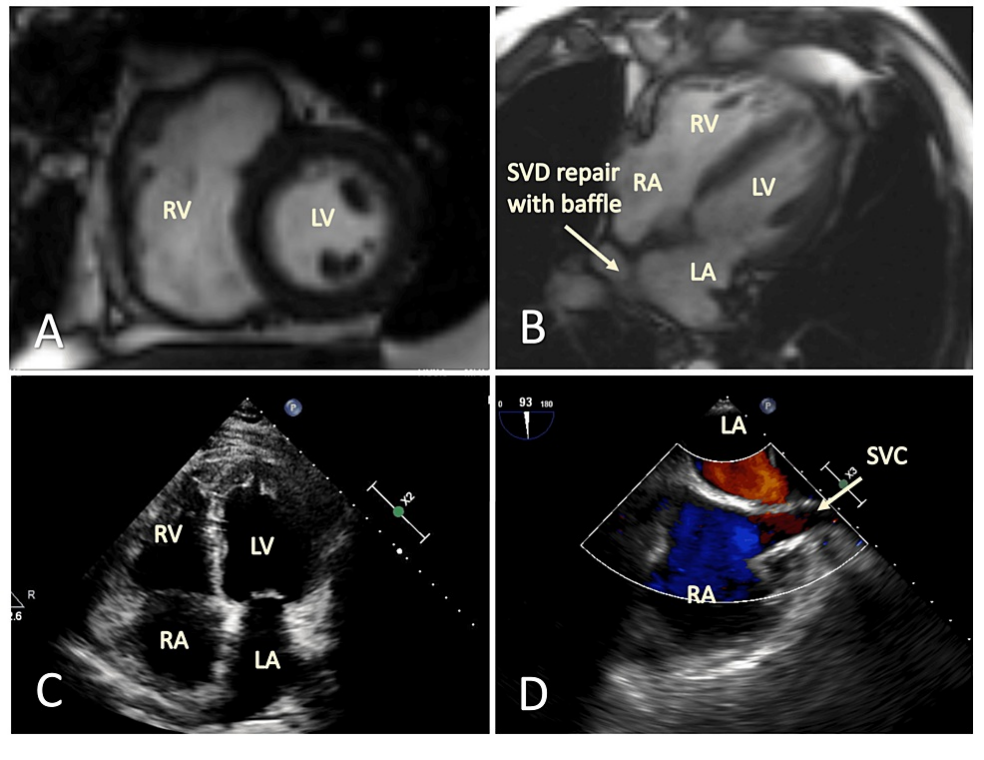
Follow-up images after surgical repair of the RSAPVR and SVD CMR images on follow-up; (A) Demonstrates an improvement in RV dilation. (B) The surgical correction of the SVD and RSAPVR repair with a baffle conduit can be appreciated, restoring the right superior pulmonary vein into the LA. (C) TTE images in the apical 4-chamber view demonstrate improved RV dilation. (D) TEE image of the bicaval mid-esophageal view demonstrates resolution of the inter-atrial shunt. CMR: cardiac magnetic resonance imaging; RV: right ventricle; RA: right atrium; LV: left ventricle; LA: left atrium; SVD: sinus venosus defect; SVC: superior vena cava; RSAPVR: right superior anomalous pulmonary venous return; TTE: transthoracic echocardiogram; TEE: transesophageal echocardiogram.
